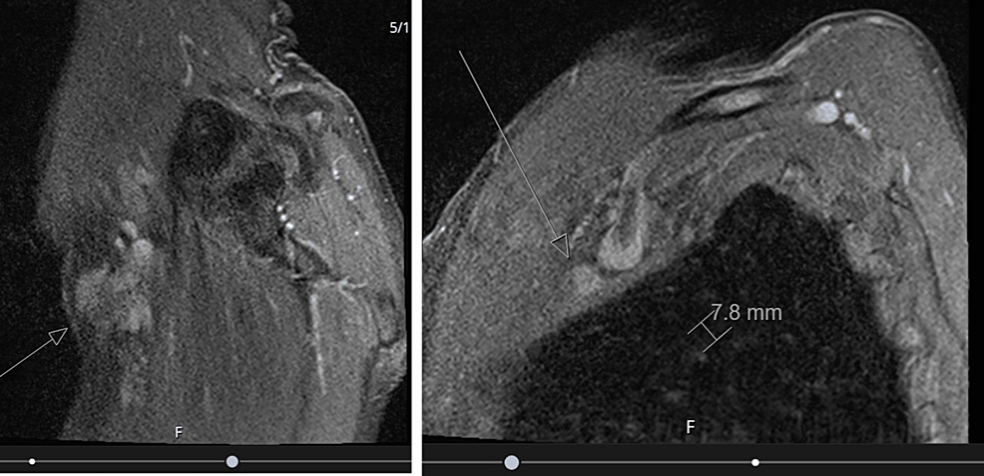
Soft tissue MRI of the chest Highlights a different section of the same image in Figure 2. Arrows indicate mass abutting the anterior head of the deltoid muscle, located immediately deep to the neurovascular structures without definite direct invasion.
